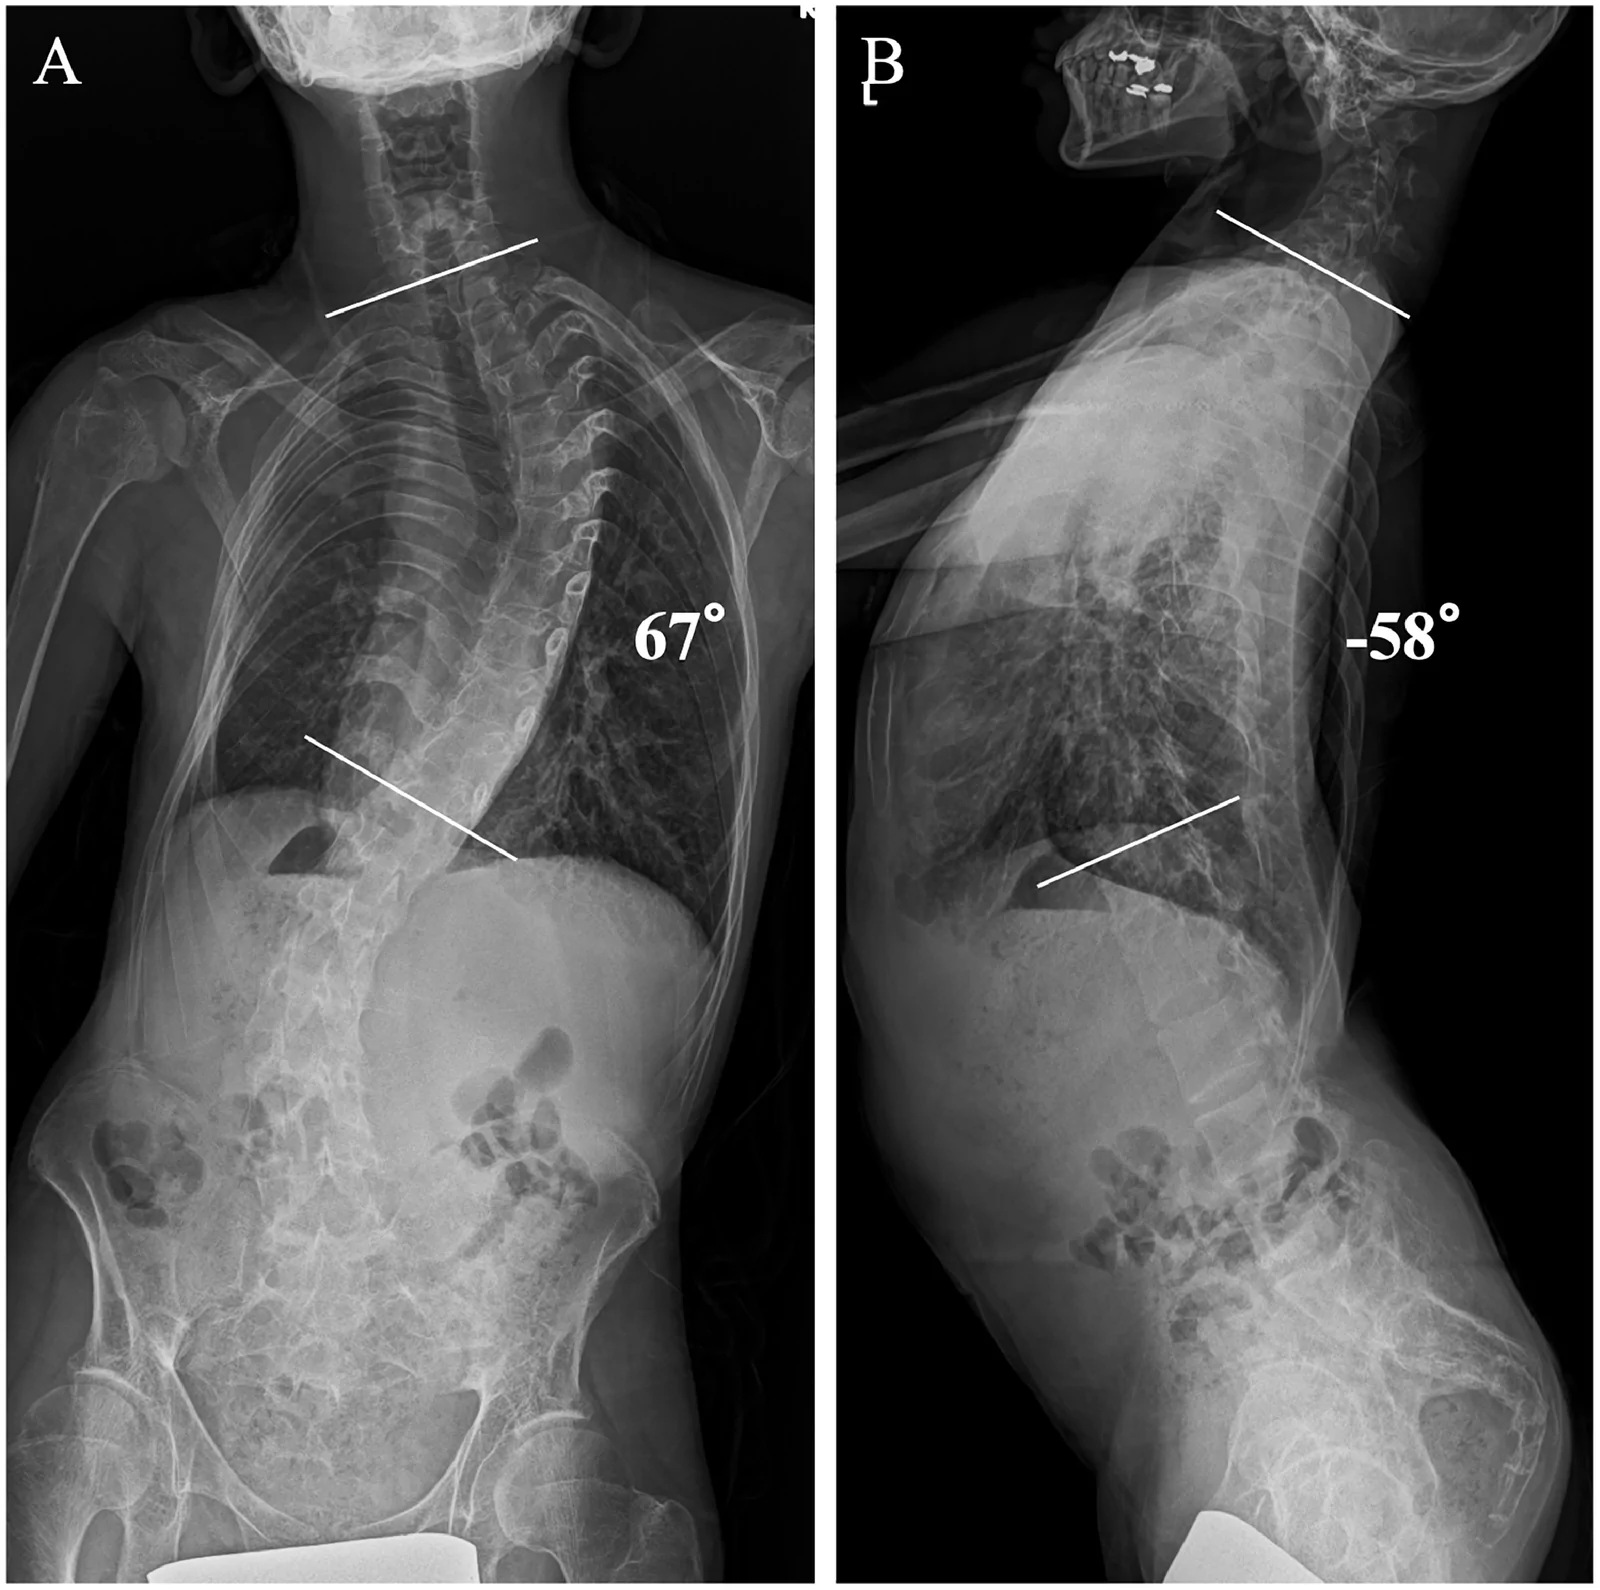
Preoperative upright whole-spine radiographs. (A) Upright anteroposterior radiograph. (B) Upright lateral radiograph. Upright radiographs showed scoliosis of 67 degrees from T1 to T12 (A) and lordosis of −58 degrees from C7 to L1 (B).
